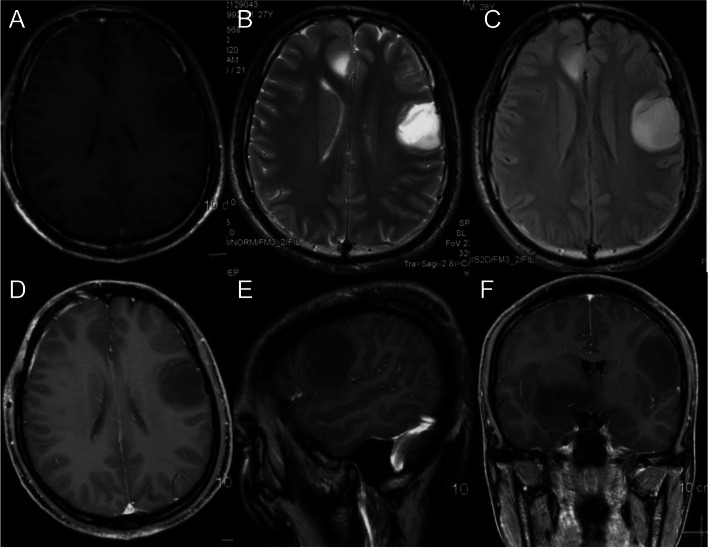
Preoperative magnetic resonance images of diffuse low-grade glioma in the left inferior frontal gyrus. A Axial T1. B Axial T2. C Axial T2 fluid-attenuated inversion recovery (FLAIR). D–F Axial, sagittal, and coronal T1 contrast
